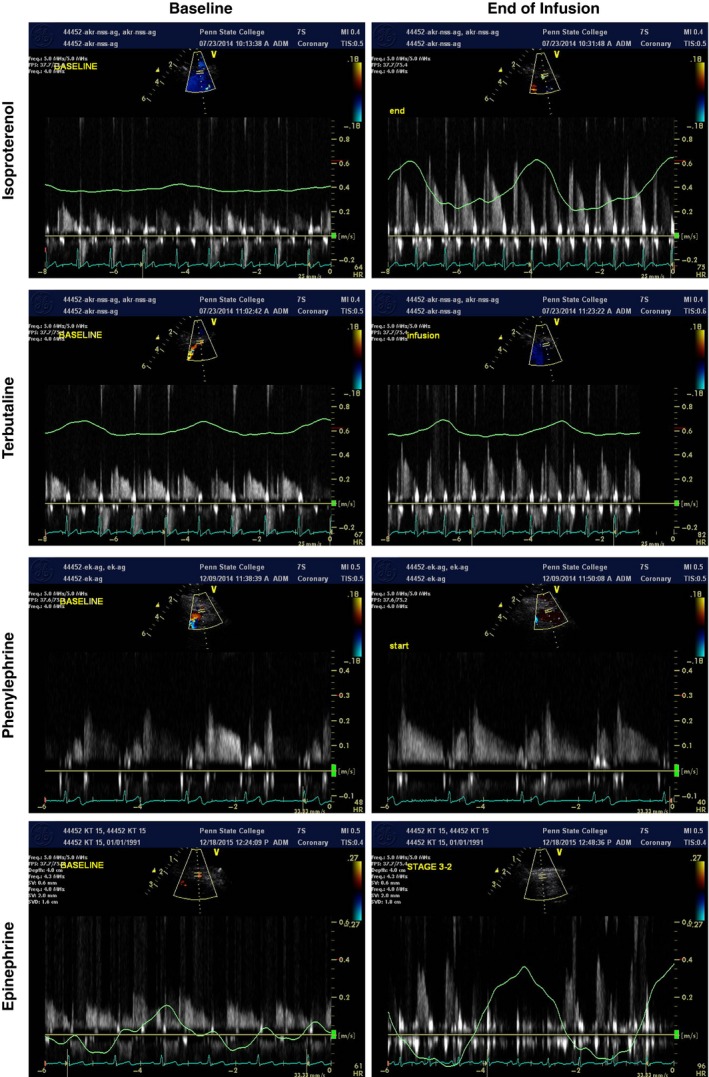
Representative echocardiographic images of coronary blood velocity (shown here in m/sec) obtained from the distal left anterior descending coronary artery during preinfusion baseline (left panels) and at the end of the agonist infusions (right panels). The peak of the diastolic velocity profile (CBVpeak) and the area under the curve (also called velocity time integral, VTI) were analyzed. The green waveform is the respiratory tracing. Please note the vertical scale is different for the phenylephrine recording.
